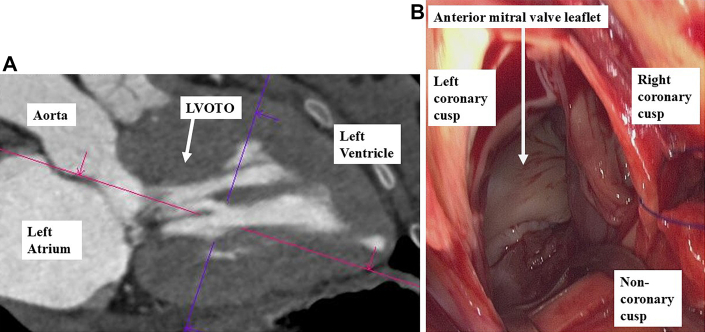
Findings Related to the LVOTO and Intraoperative View From the Aortic Valve Approach (A) Preoperative CT showing left ventricular hypertrophy and a suspected obstructive lesion causing LVOTO. (B) The aortic valve was rotated >30° clockwise, and the right noncoronary commissure was located far from the mitral valve. CT = computed tomography; LVOTO = left ventricular outflow tract obstruction.
